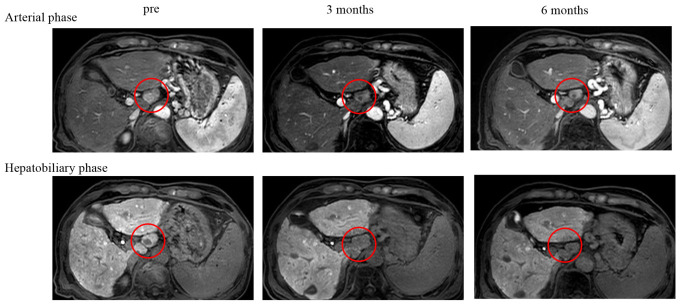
Representative gadolinium ethoxybenzyl diethylenetriamine pentaacetic acid-enhanced MRI demonstrating complete response. Arterial and hepatobiliary phase images before and at 3 and 6 months after SBRT are shown. At 3 months after SBRT, disappearance of arterial phase enhancement is observed (circle), consistent with complete response according to modified RECIST criteria.
